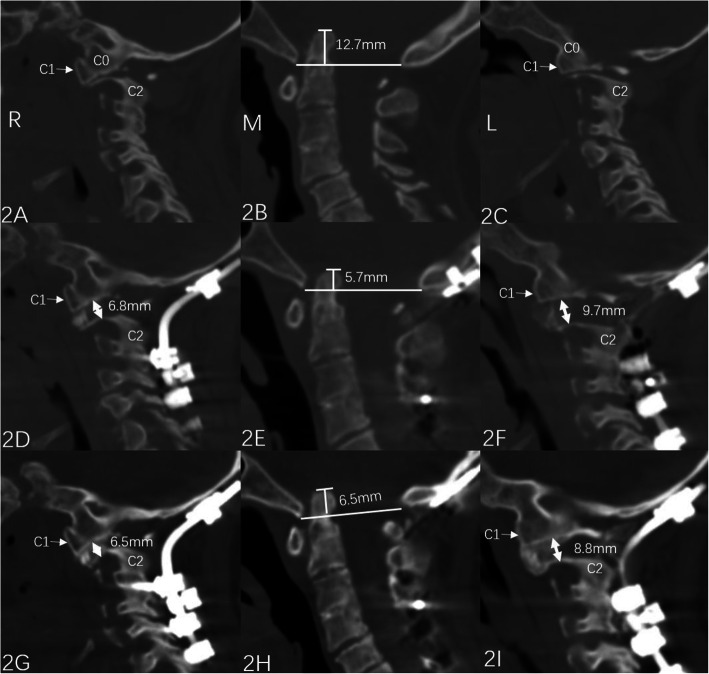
A rheumatoid arthritis with BI managed with posterior structural fusion. Female, 41 years. She reported a history of rheumatoid arthritis for 16 years. Symptoms of myelopathy lasted for 1 year (JOA score 9). She underwent occipital-C4 fixation, release of the atlantoaxial facets, and intraarticular structural fusion. Her JOA score improved to 15 at the final follow-up. A–C Preoperative CT slice: The right atlantoaxial facet joint (2A), midsagittal (2B), and left atlantoaxial facet joint (2C) views showed the relationship among C0, C1, and C2. The distance from the tip of the odontoid process to Mcrea’s line was 12.7 mm, and the secondary BI was due to RA-induced collapse of the lateral mass column. D–F Immediate postoperative CT slice: Structural grafts were inserted into facet joints with heights of 6.8 mm on the right side (2D) and 9.7 mm on the left side (2F, white double arrow), and the BI was lessened to 5.7 mm (2E). G–I CT at 5 months following surgery: Both the BI correction (6.5 mm, 2H) and the height of the joint space (6.5 mm at right, 2G; 8.5 mm at left, 2I) were maintained well, and robust interfacet fusion was acquired
